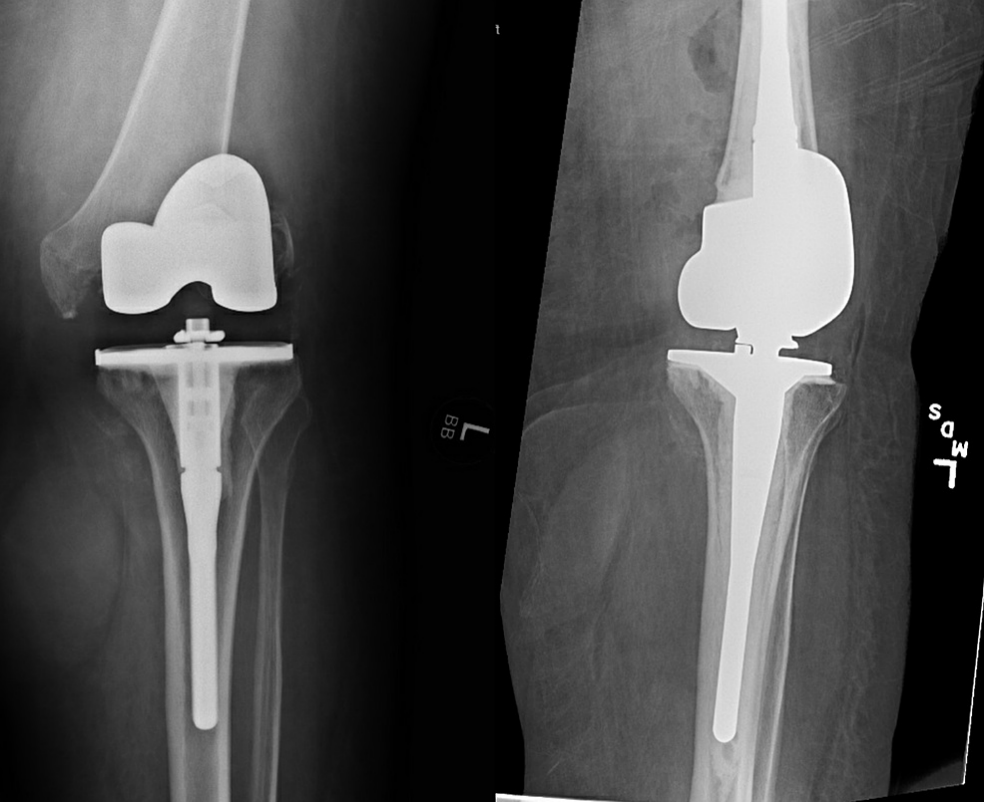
Readmission radiographs post primary total knee arthroplasty showing a periprosthetic distal femur fracture (left); status post revision total knee arthroplasty due to periprosthetic distal femur fracture (right)
